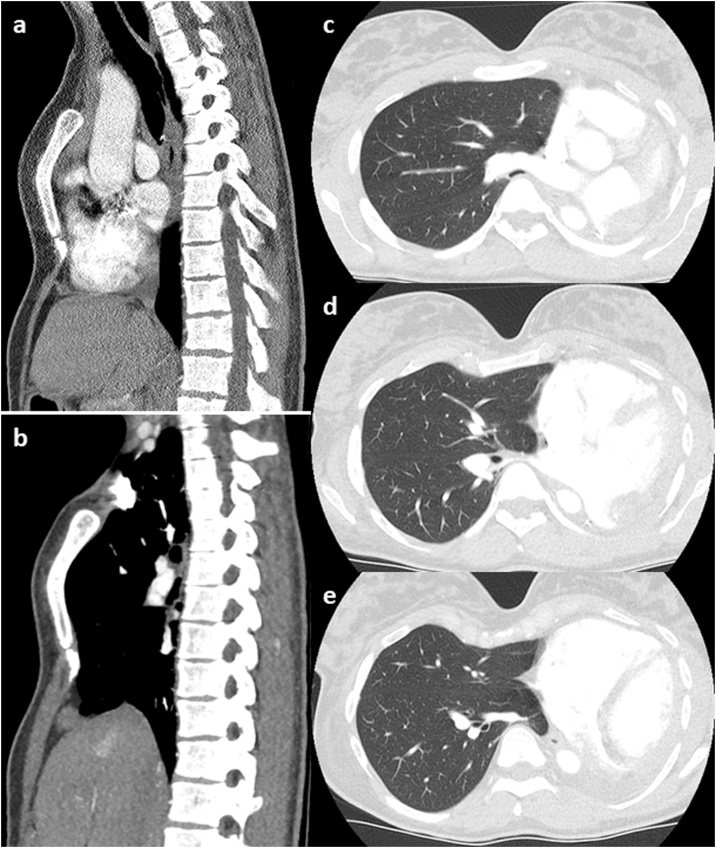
CT status of postpneumonectomy syndrome 6/2019. a) Sagittal CT-scan 2017 demonstrating pectus excavatum prior to pneumonectomy. b) Sagital CT-scan 6/2019 with complete rotation of the heart into the left thoracic cavity. c–e) CT-scans showing the reasons for postpneumonecotmy complains: compression of the right main bronchus posterior to the right pulmonary artery (c), stretching and compression of the right lower pulmonary vein inferior to the right main bronchus (d), minimum distance between posterior border of the sternum und anterior border of the column, not allowing simple repositioning of the heart retrosternally (e).
